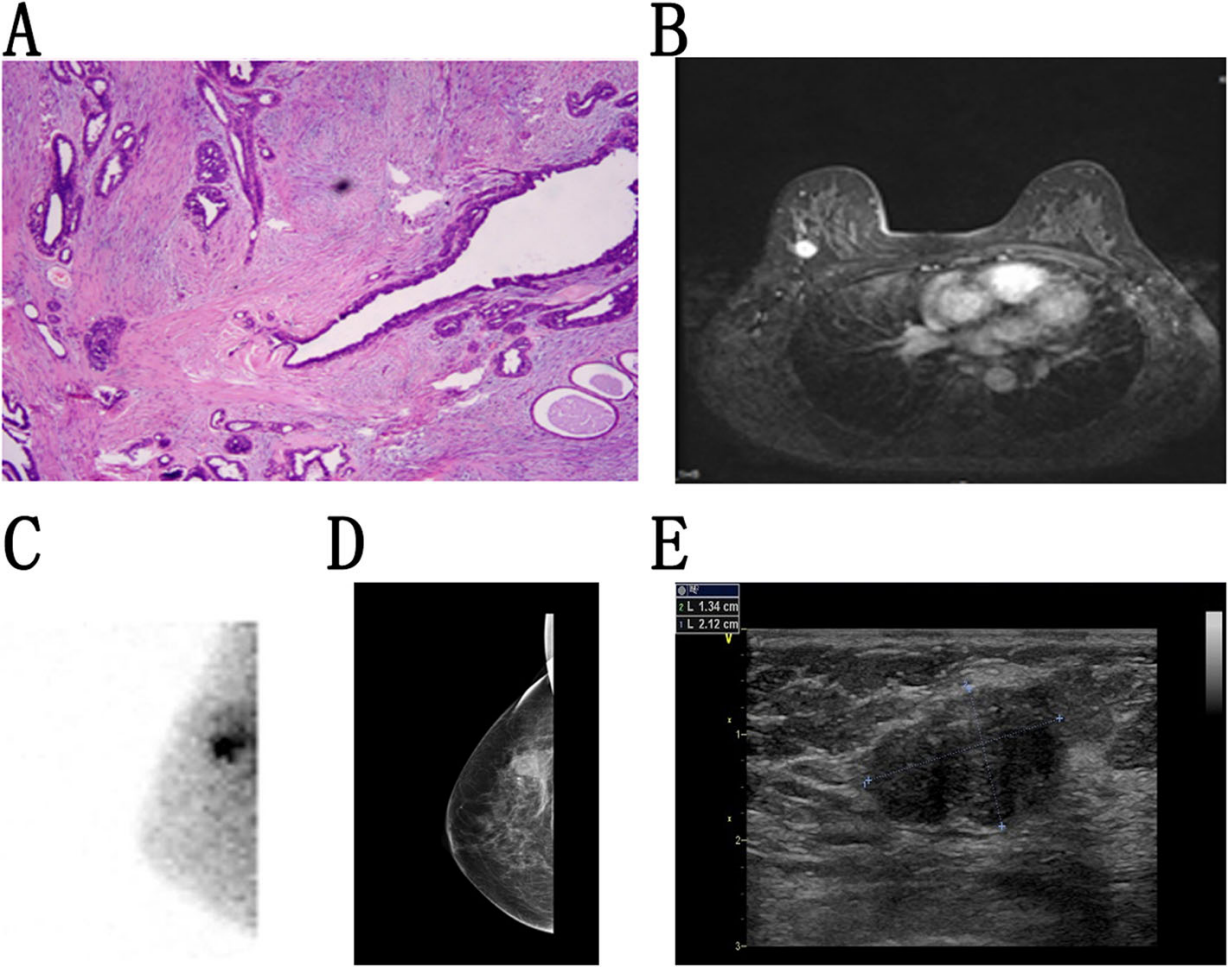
Breast images from a woman. a. Pathology identified a fibroadenoma. b. MRI results revealed a nodular mass (BI-RADS 4C) in the right breast,1.9 × 1.5 cm. c. BSGI revealed focally increased radiotracer uptake in the right breast that was consistent with malignancy, LNR = 2.49. d. Mammography demonstrated a high-density shadow (BI-RADS 4C) in the upper outer region of the right breast, 1.3 × 1.8 cm. e. Ultrasound revealed an elliptical hypoechoic mass (BI-RADS 4C) in the right breast, 1.3 × 2.1 cm
